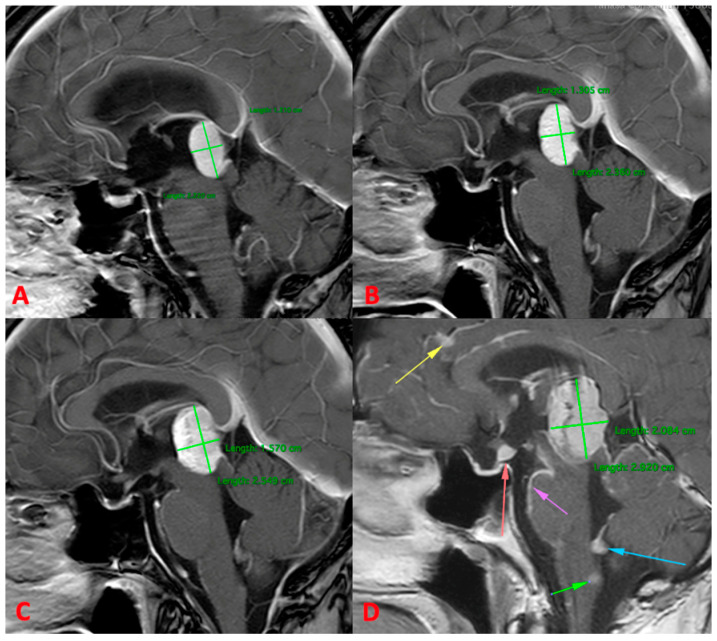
MRI images showing the evolution of the lesion from the diagnosis (A) and after radiation therapy at 4 months (B) and 12 months (C), showing moderate progression and (D) MRI at 16 months, showing important growth of the lesion and leptomeningeal spreading with multiple intracranial metastases (arrows).
